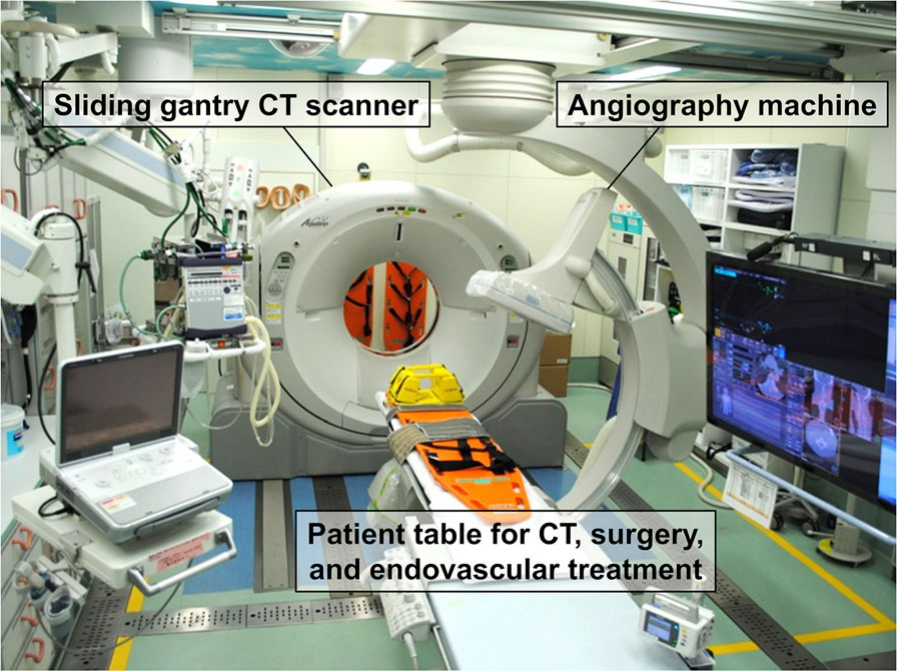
Picture of the angiography-CT equipment installed in the hybrid ER. CT scanning, REBOA, emergency surgery, and endovascular treatments are performed in this room without patient transfer. CT, computed tomography; ER, emergency room; REBOA, resuscitative endovascular balloon occlusion of the aorta
